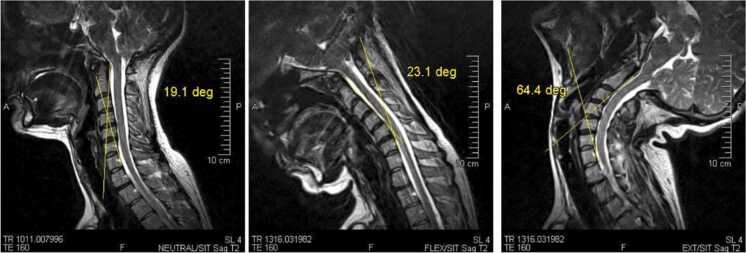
Upright MRI demonstrating load-dependent stenosis and cord compression compared with supine imaging.
